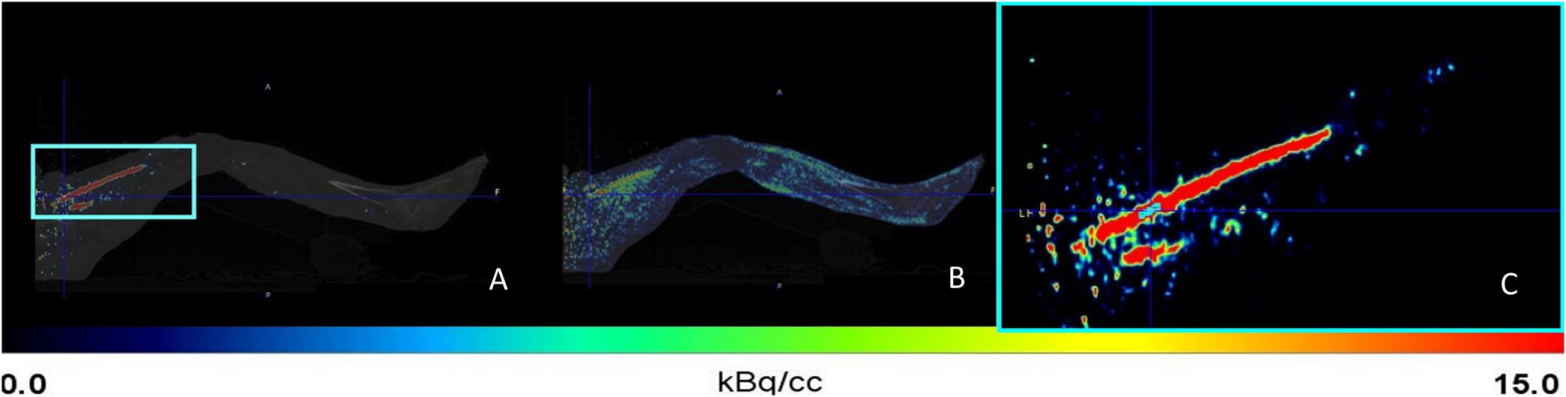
Sagittal planes of the left leg from the first (preintervention) scan of the first patient. (A) Fusion of positron emission tomography (PET) and computed tomography (CT) scan at an early time frame (frame 5; 19 s). (B) Fusion of PET and CT scan at a late time frame (frame 22; 309 s). The turquoise box in panel A highlights the superficial femoral artery (SFA), located anterior to the adductor magnus muscle and posterior to the vastus medialis, corresponding to the area used for further analysis and shown in greater detail in panel C. Turquoise = region used for arterial time–activity curves.
